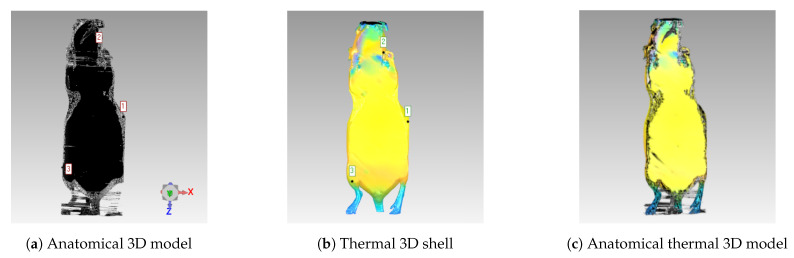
Registration Process: (a) 3D model generated from anatomical CT images. (b) Thermal 3D model. (c) Registration of both 3D models, generating the complete model (visualized using the same coordinate system).
